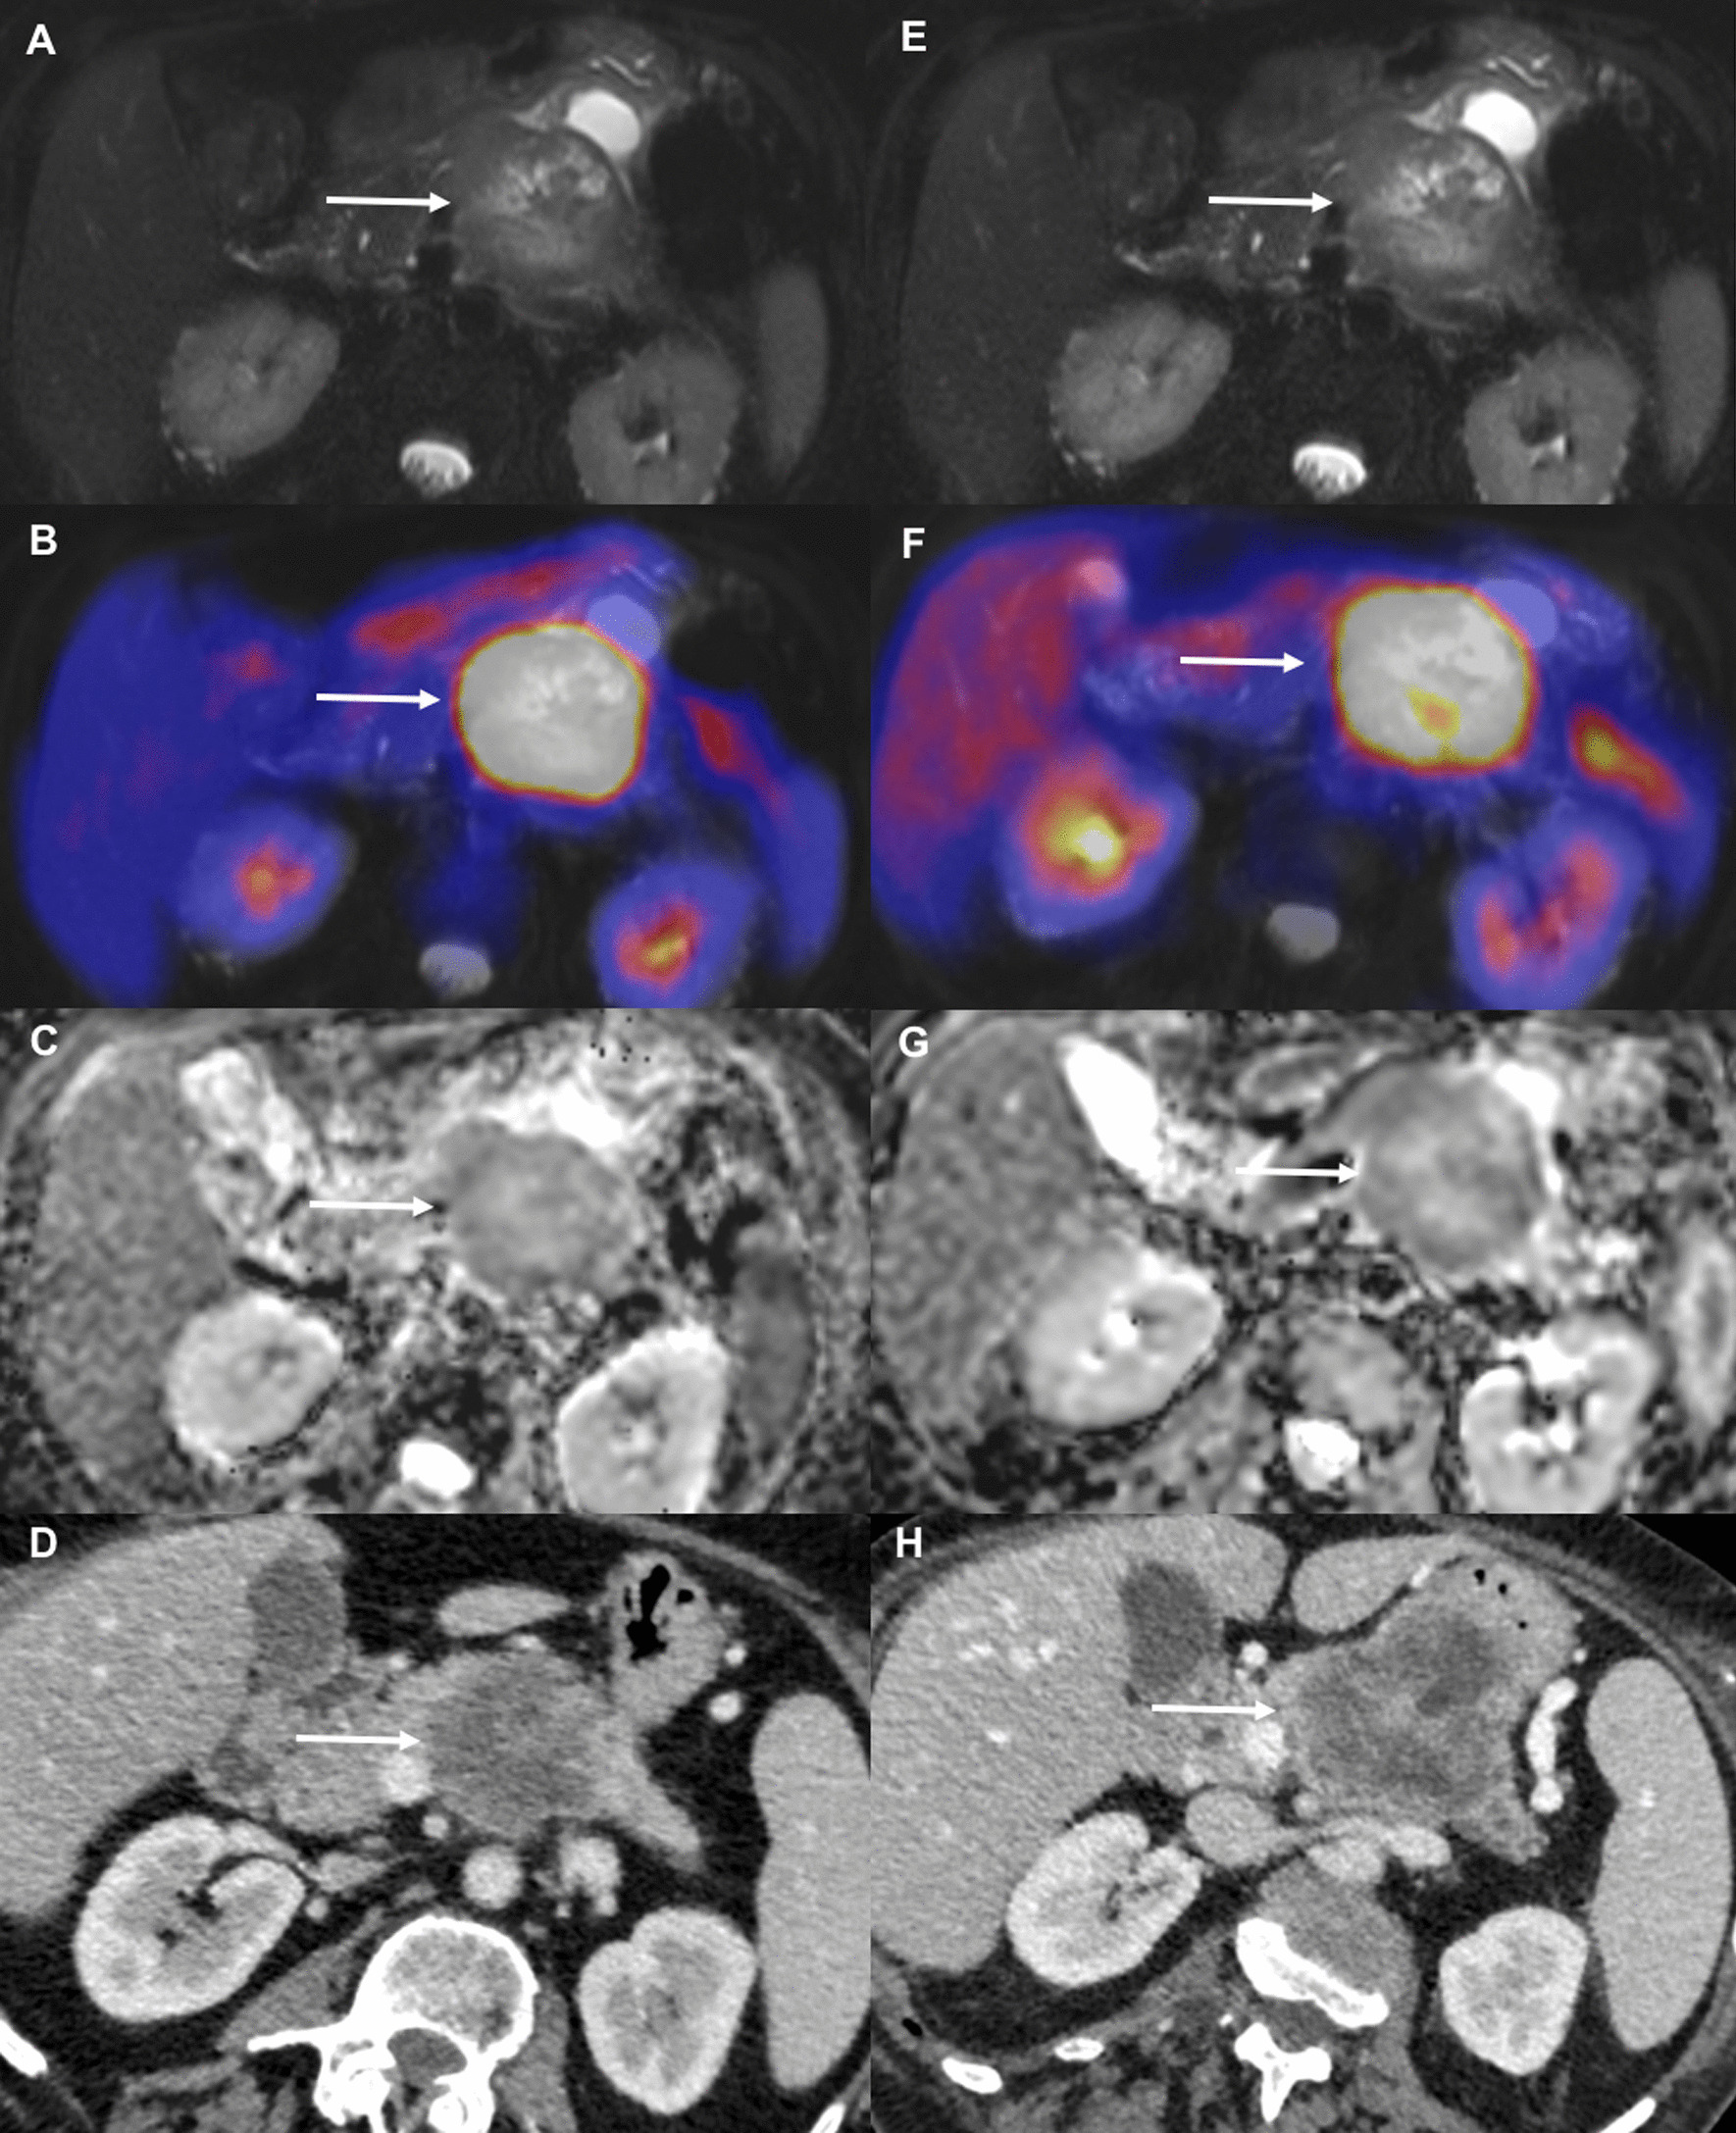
Images from a 66y-old female patient with a PDAC in the pancreatic corpus. Images on the left (a–d) were obtained prior to chemotherapy, images on the right (e–h) were obtained 14 days later, after one cycle of palliative chemotherapy with FOLFIRINOX. Axial T2 FS images (a, e) display no significant change in tumor size. However, PET showed a significant increase in MTV_50%_ (33.8%) and TLG_50%_ (19.9%) (b, f). Additionally, only a slight increase in ADC_mean_ (7%) was seen (c, g). The follow-up CT (h) after 78 days reveals progressive disease despite 6 cycles of FOLFIRINOX compared to the baseline CT (d)
